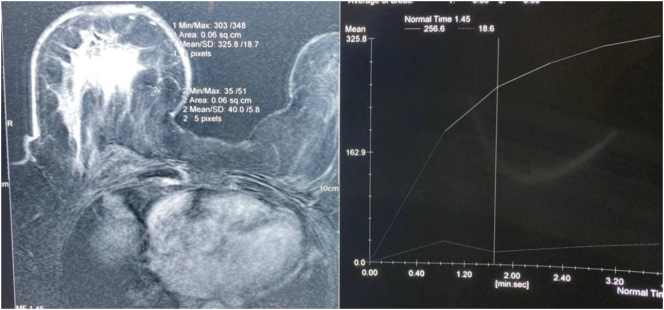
Magnetic resonance imaging (MRI) with plotting showing diffuse homogeneous parenchymal enhancements with diffuse tranecular and skin edema (high signal on Short-TI Inversion Recovery (STIR)), after plotting Total Ion Chromatogram (TIC) to the enhanced parenchyma, showing initial and persistent raising delay phase (type I curve).
